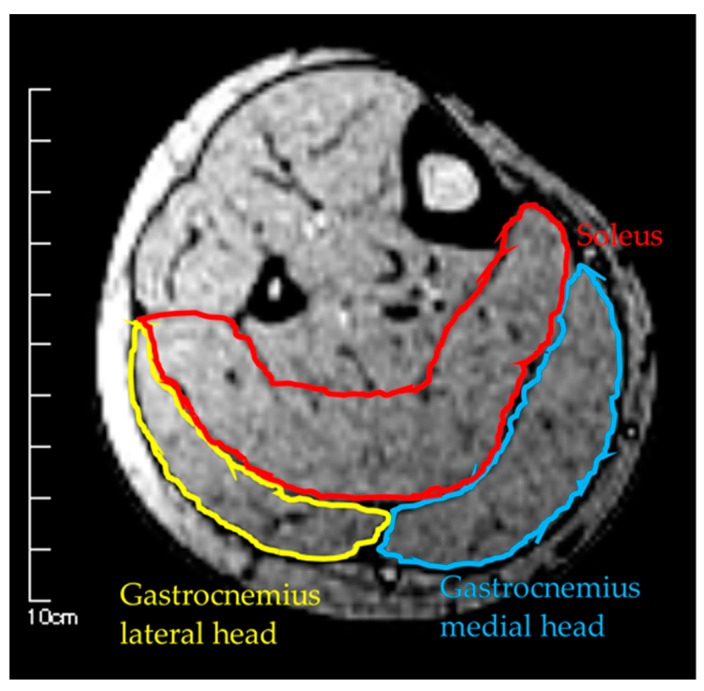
Measurement of muscle cross-sectional area by MRI.
